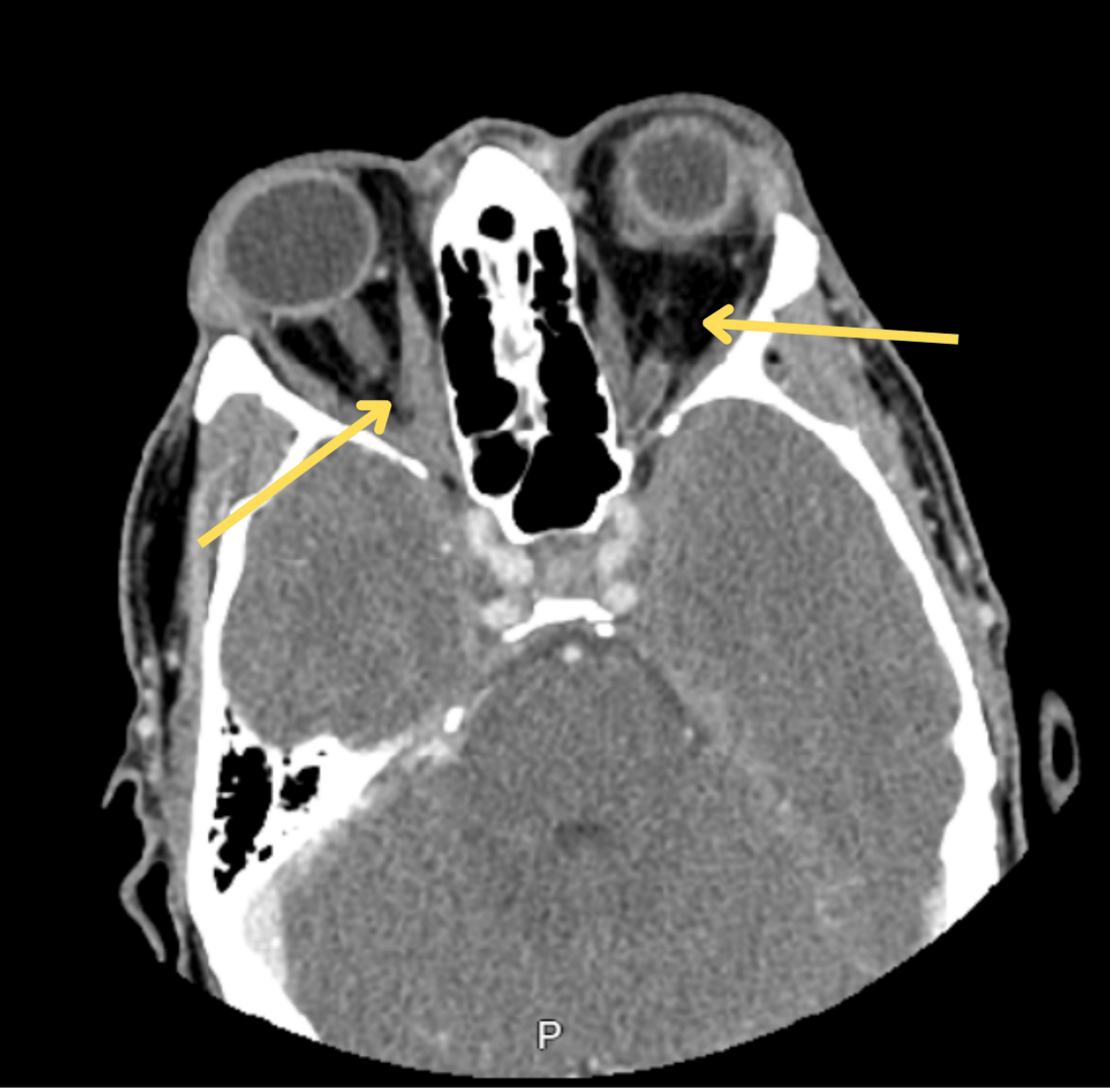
CT orbit showed bilateral proptosis with slight thickening of the muscle bellies of the superior, medial, and inferior rectus musculature with adjacent fat stranding
